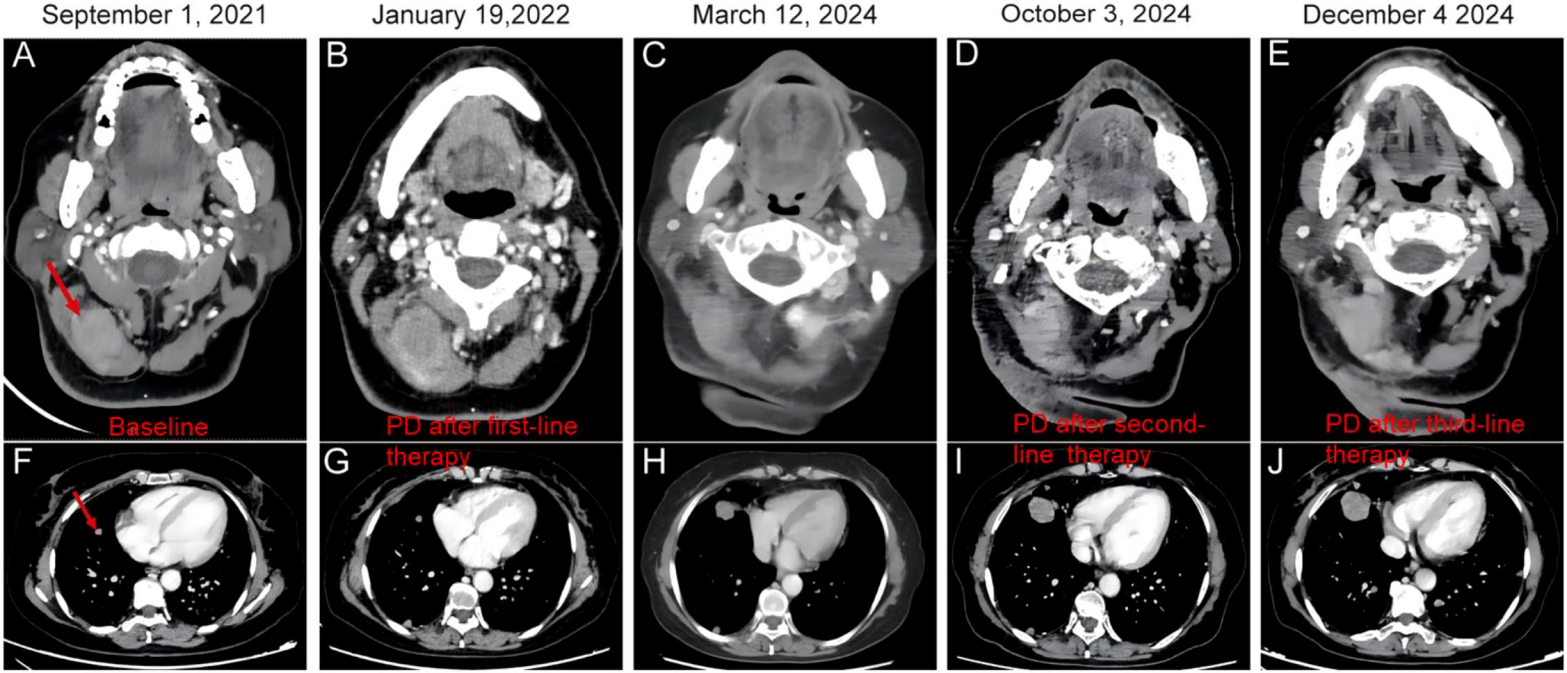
Computed Tomography (CT) images of a 54-year-old female with recurrent malignant melanotic nerve sheath tumor with multiple lung metastases. Serial CT scans of the neck (A–E) and chest (F–J) demonstrate persistent imaging abnormalities over time. The red arrow showed irregular soft tissue masses in the right posterior neck (A-E). The red arrows show soft tissue density nodules of different sizes in the lungs, which show mild to moderate enhancement in the enhanced phase (F-J).
